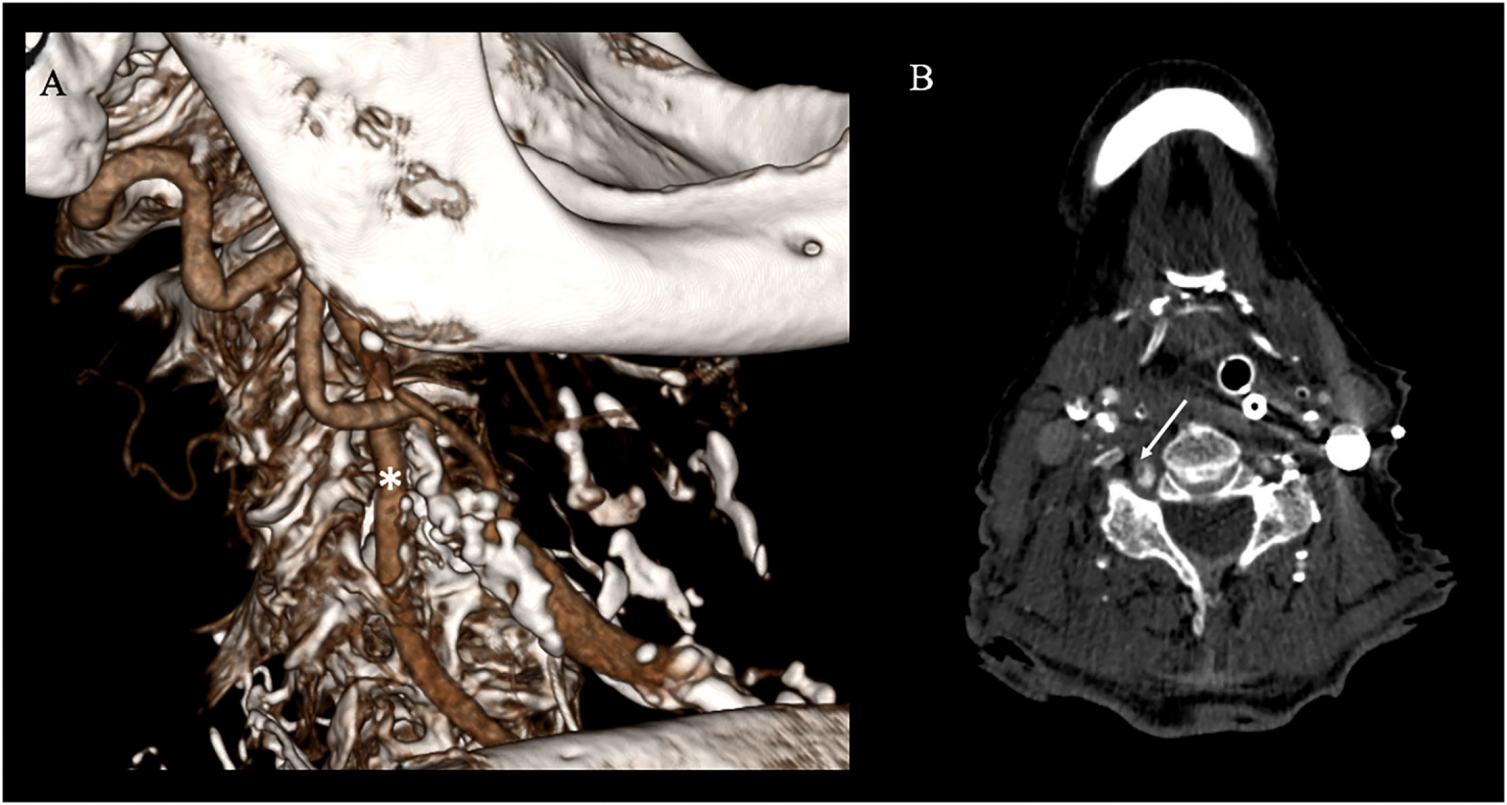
Follow-up vascular imaging. (A) CT-angiography (CTA) with sagittal 3D-reconstruction shows continuous contrast filling of the vertebral artery (*; white star) and no vascular. (B) However axial CTA shows evidence of a residual wall adherent thrombus at the former access site section but no cervical hematoma (white arrow).
